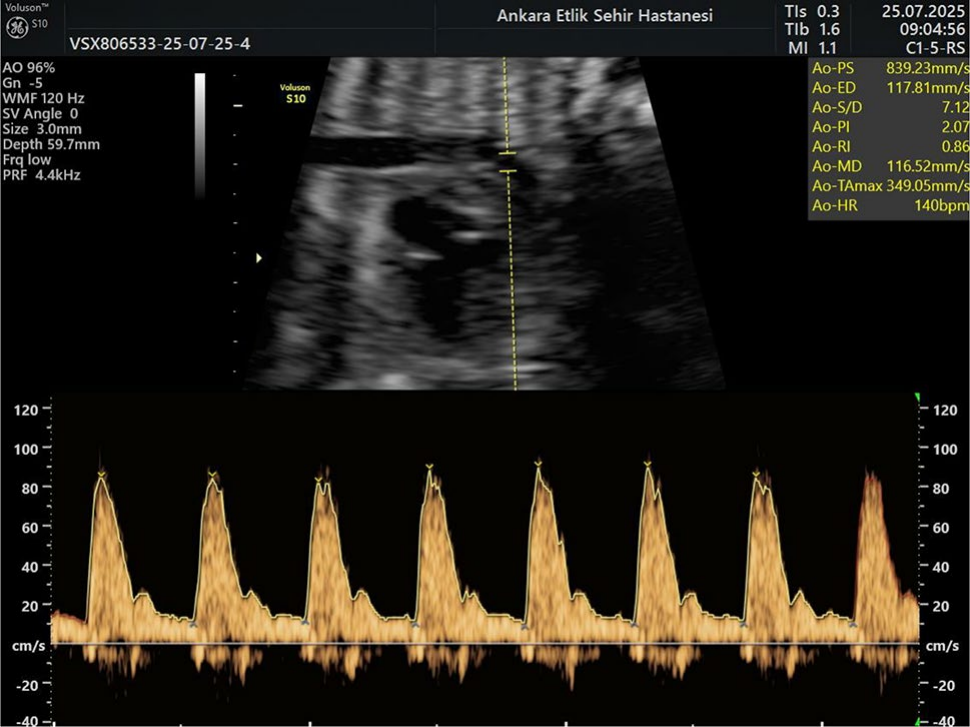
Pulsed-wave Doppler assessment of the fetal aortic isthmus showing forward flow during systole and early diastole in a sagittal view
